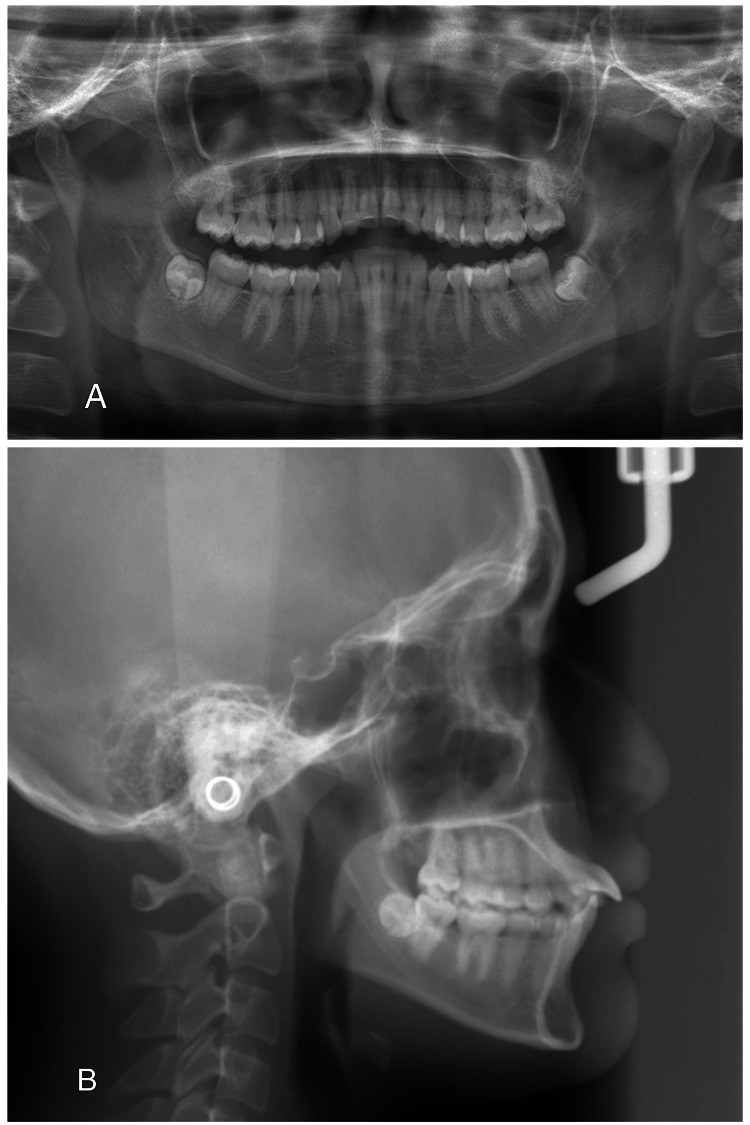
Pre-treatment radiographs: (A) panoramic radiograph and (B) lateral cephalogram
